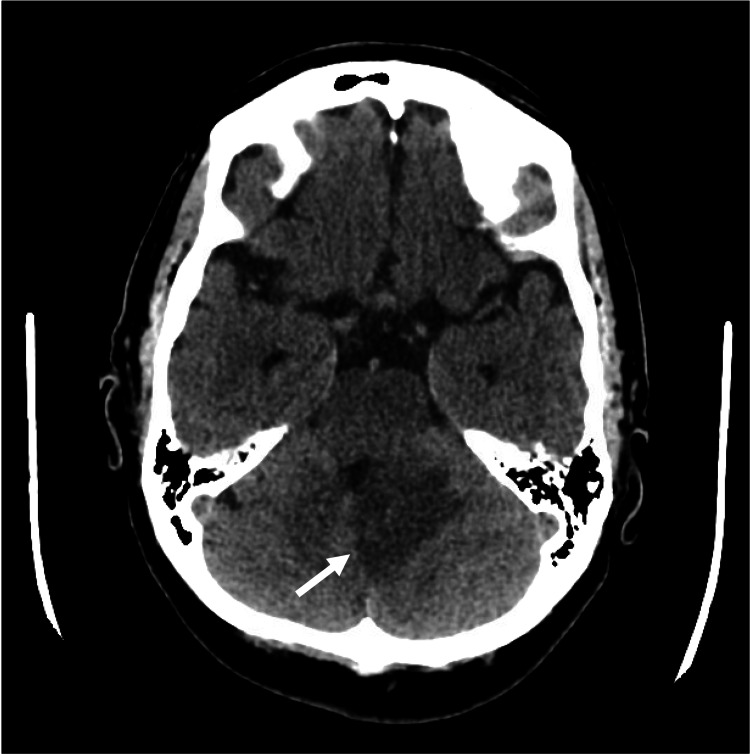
CT head demonstrating left cerebellar vasogenic edema with mass effect on the fourth ventricle.
